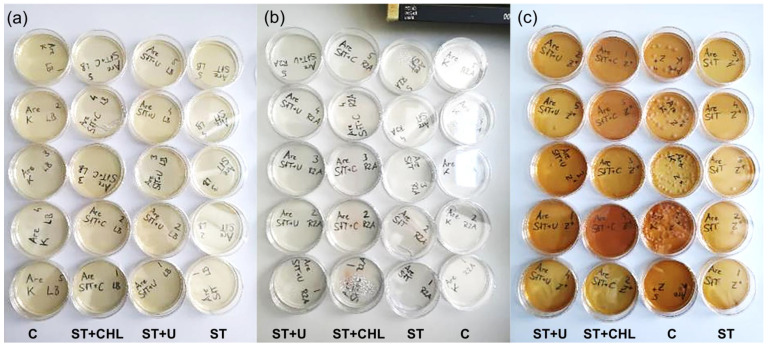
Plates 3 days after inoculation: (a) No colonies are visible for any of the treatments on LB medium. (b) In R2A medium, a few bacterial colonies were monitored for all treatments with highest contaminations in controls (red clusters). (c) Contamination was confirmed across all treatments on agar plates with Zarrouk+ medium. Abbreviations indicate steps to reach axenicity: C = control without dilution, ST = standard treatment, ST + CHL = standard treatment + chloramphenicol, and ST + U = standard treatment + ultrasonication.
